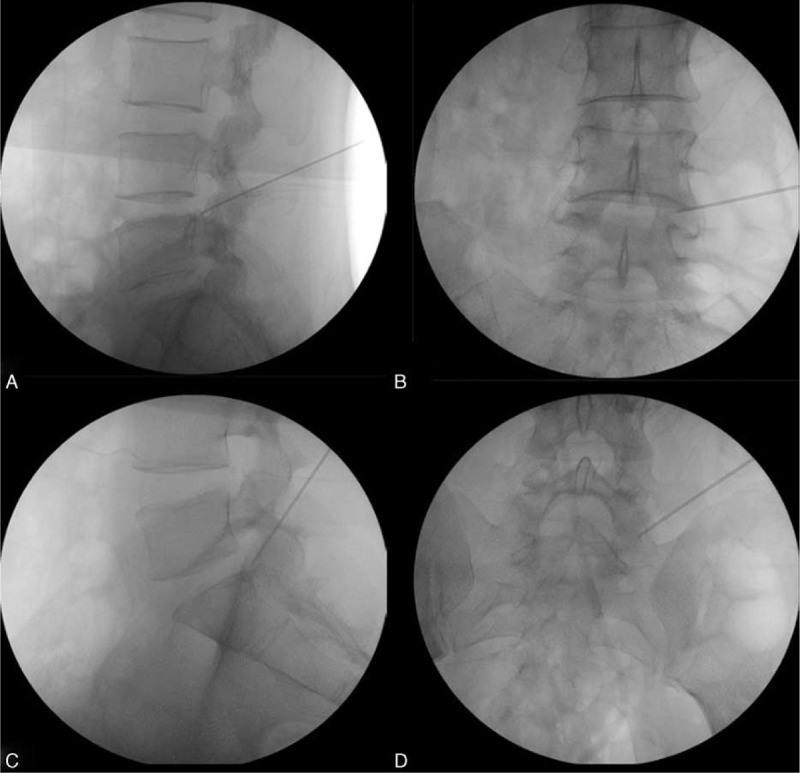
Intraoperative fluoroscopic images showing the modified technique (superior border of inferior pedicle approach), the downward inclination of the needle trajectory with the needle passing through the superior border of inferior pedicle on the lateral radiograph and the needle tip lying at the medial pedicular line on the anteroposterior radiograph (The upper pictures showing the approaching at L4/5 disc level, and the bottom pictures showing that at L5/S1 disc level).
